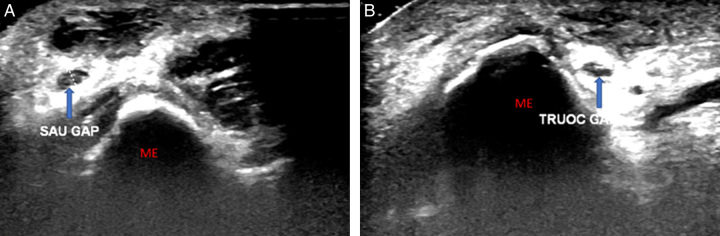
Dynamic ultrasound evaluating the ulnar nerve’s position, (A) over than 90° elbow flexion, (B) elbow extension, (blue arrow) ulnar nerve. ME, medial epicondyle.
